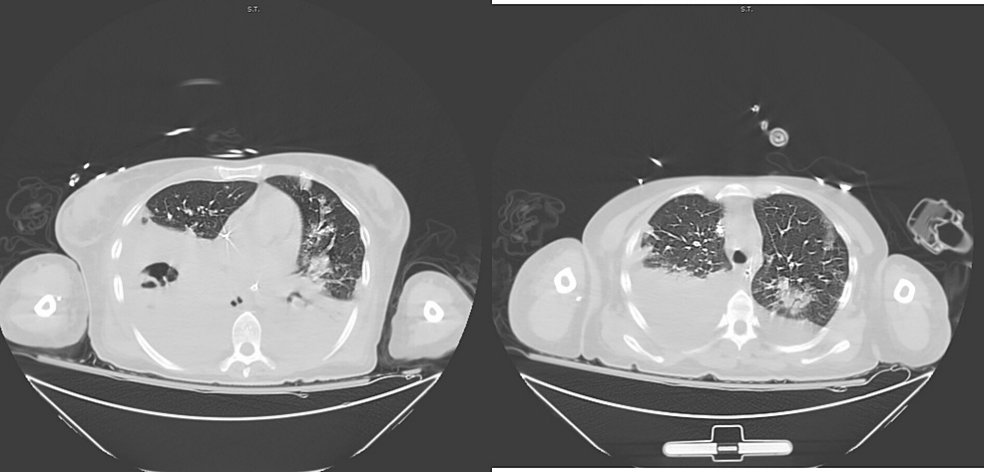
Chest CT. Left: Chest CT suggestive of necrotizing pneumonia with possible abscess on the right lung. Right: Chest CT showing diffuse multifocal nodular airspace disease.
